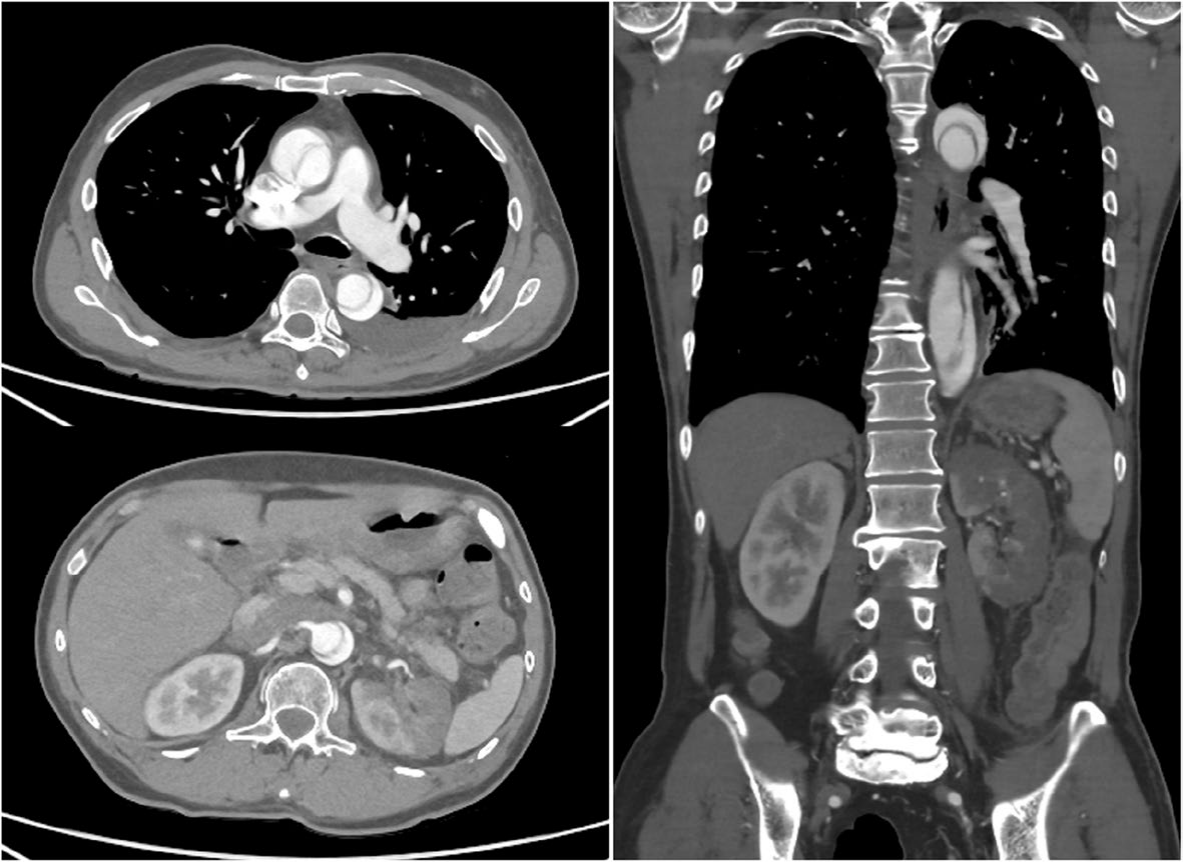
Preoperative contrast-enhanced computed tomography. The aortic dissection extends from the aortic root to the distal aorta. A left renal infarction is also present
